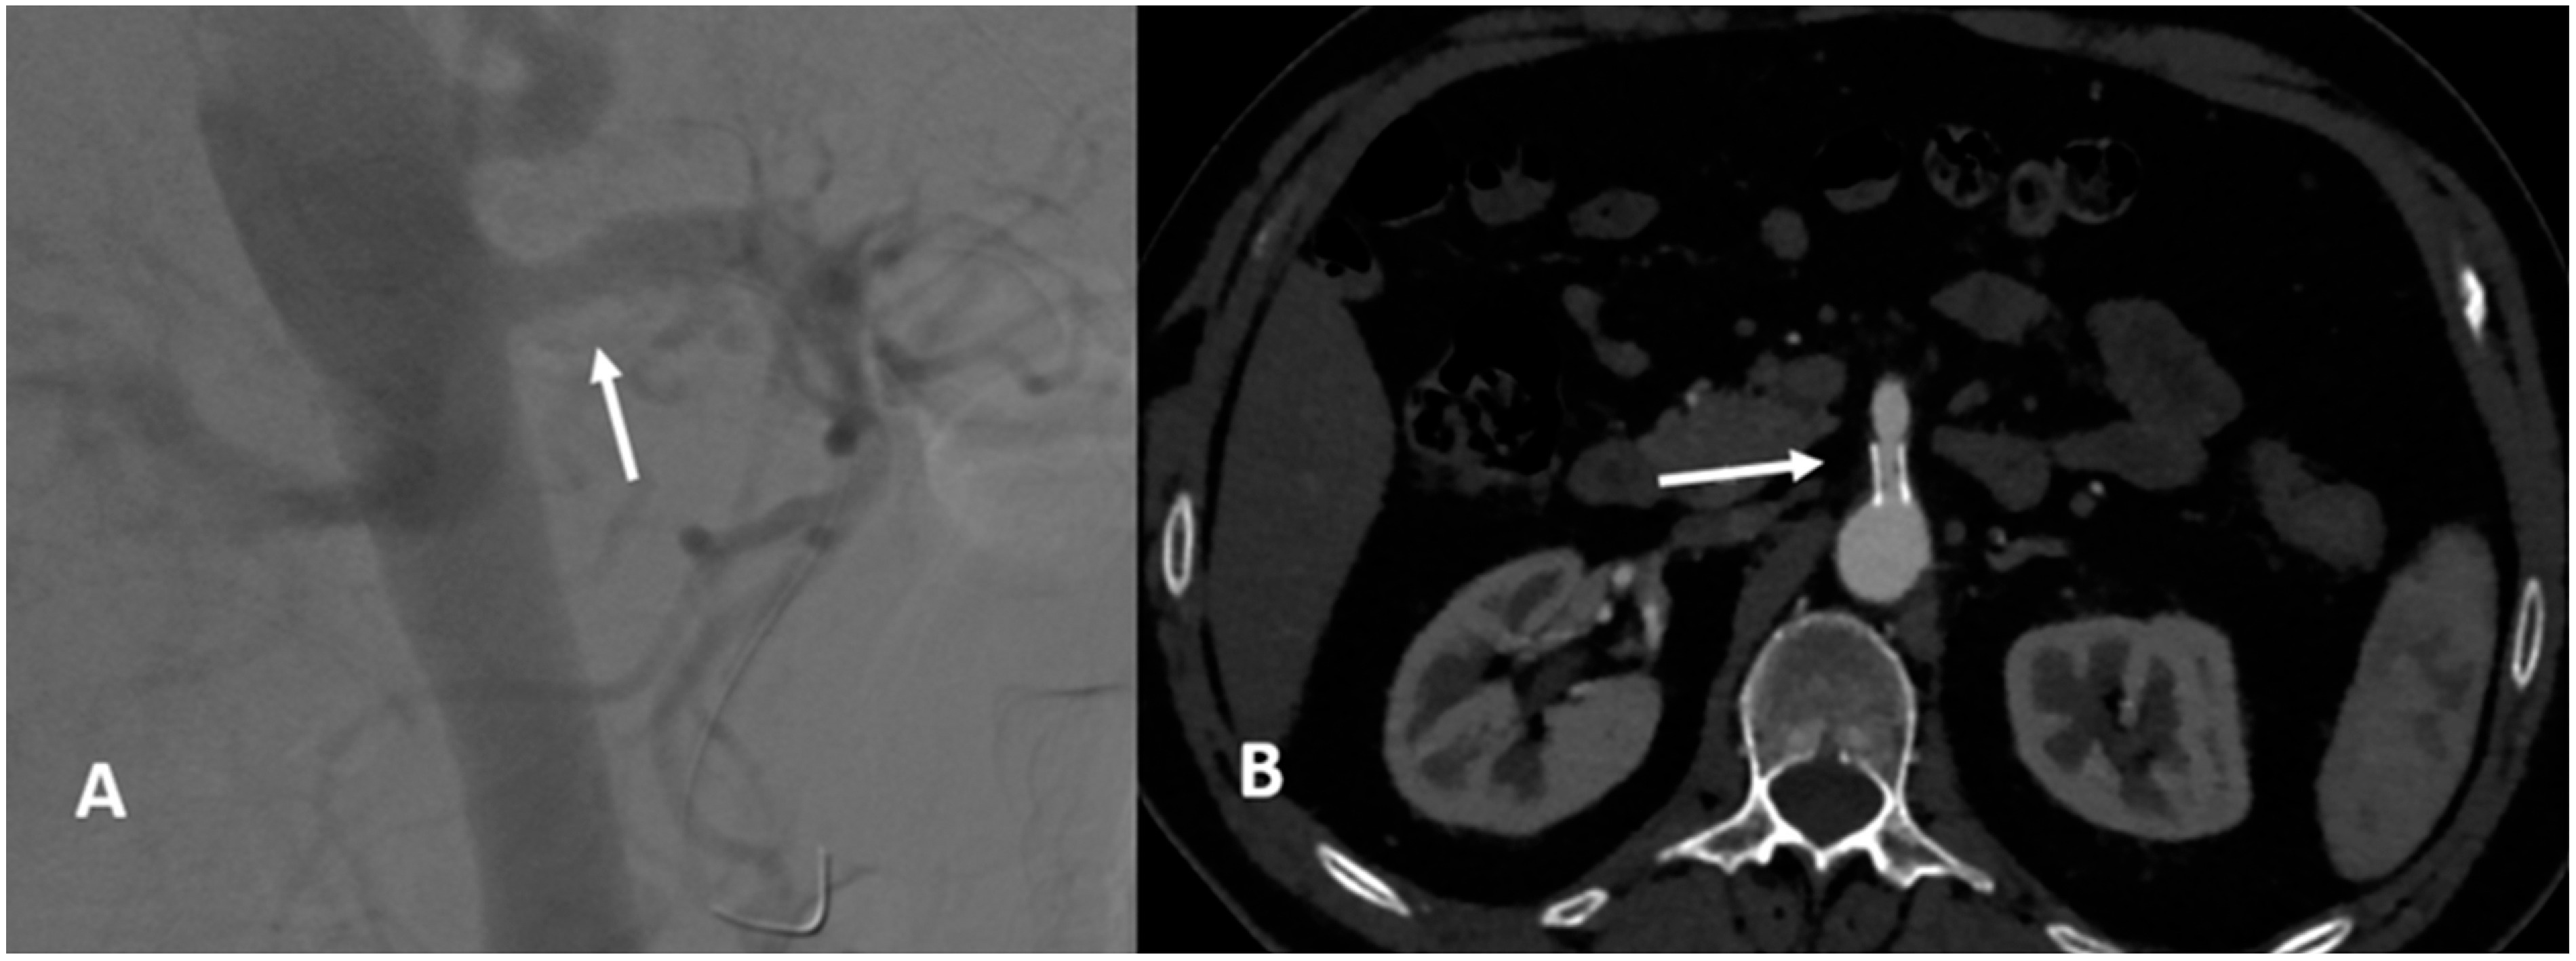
Fig. 3 (A) Angiography post-stenting showing patent superior mesenteric artery (SMA). (B) computed tomography angiography 6 months post repair. The SMA is patent, but there is evidence of minimal intimal hyperplasia.
